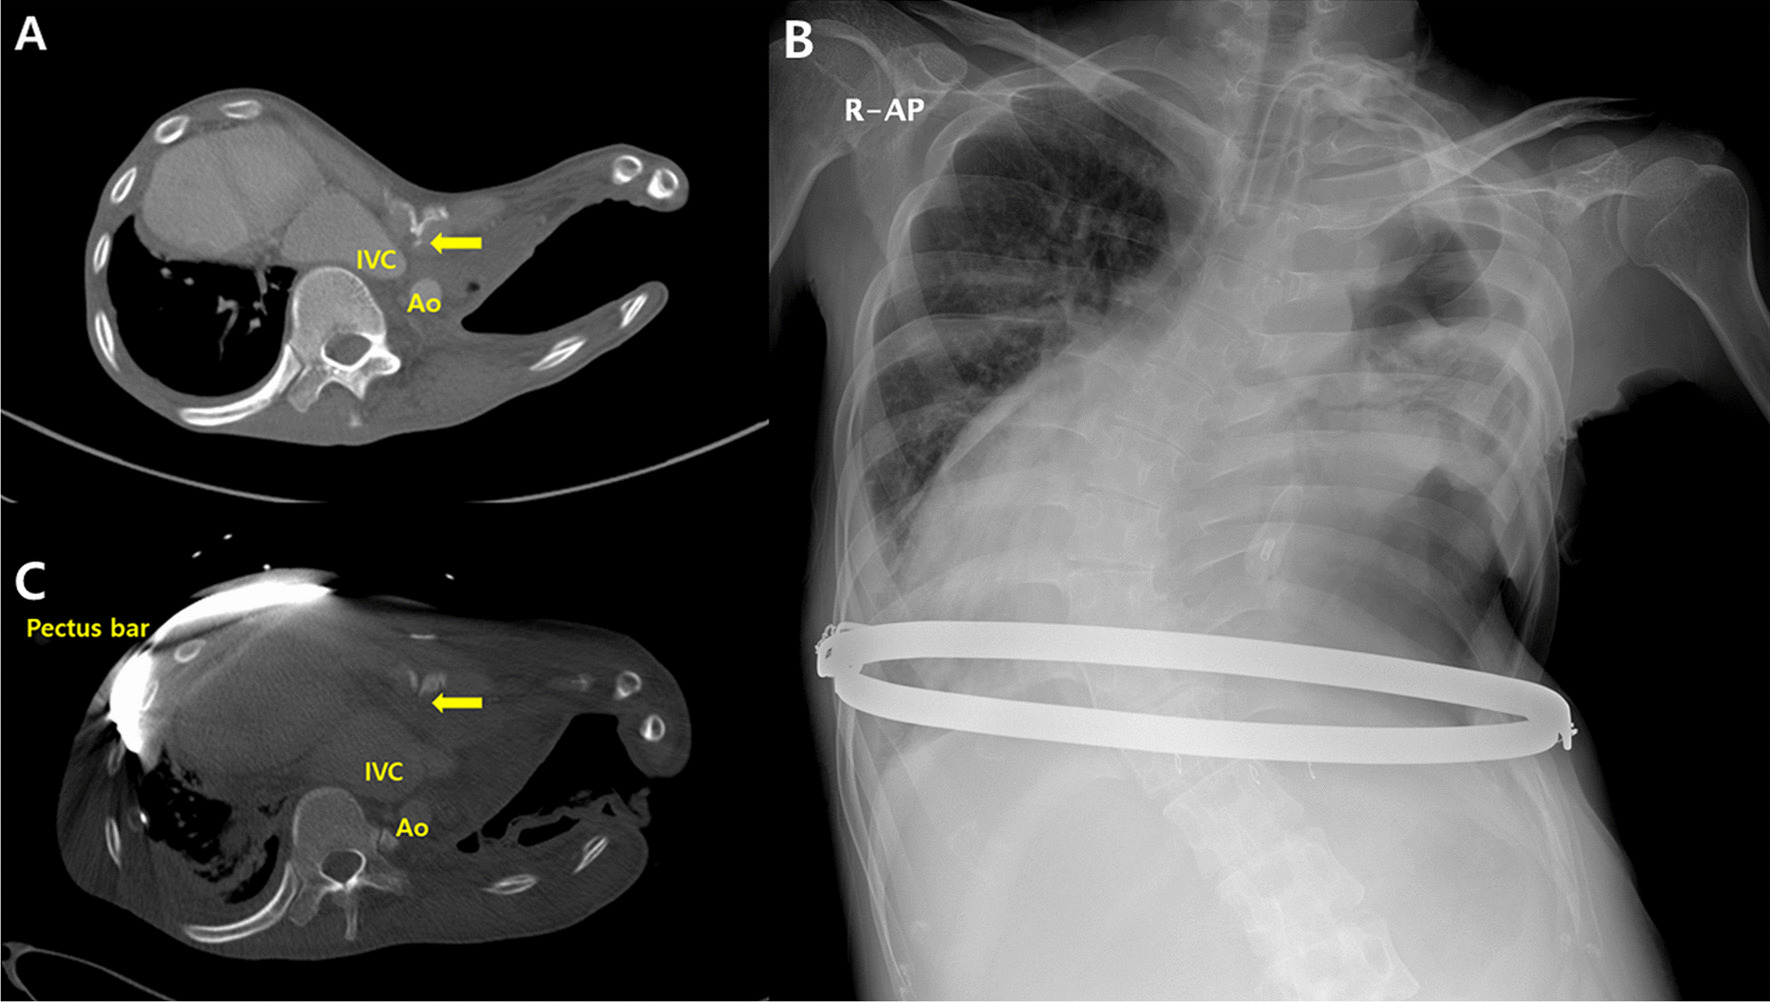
Images before and after the extrapleural Nuss procedure. A Initial chest computed tomography shows severe chest wall depression, compression of the inferior vena cava, spinal scoliosis, dextrocardia, and chronic left empyema with the Eloesser window. B A simple chest x-ray at discharge shows the extrapleural Nuss procedure with the sandwich technique. The patient had a tracheostomy for ventilator support. C A one-month postoperative chest computerized-tomography reveals a marked relief of compression on the heart and inferior vena cava by the depressed chest wall (IVC: inferior vena cava, Ao: descending aorta, arrow: most depressed point)
